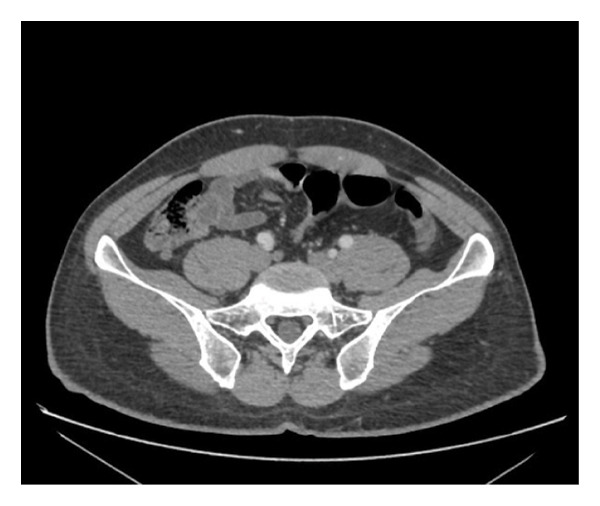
A contrast‐enhanced CT scan of the abdomen and pelvis revealing a nonaerated, fluid‐filled structure predominantly at the base of the appendix, without surrounding fat stranding or evidence of acute inflammation.
